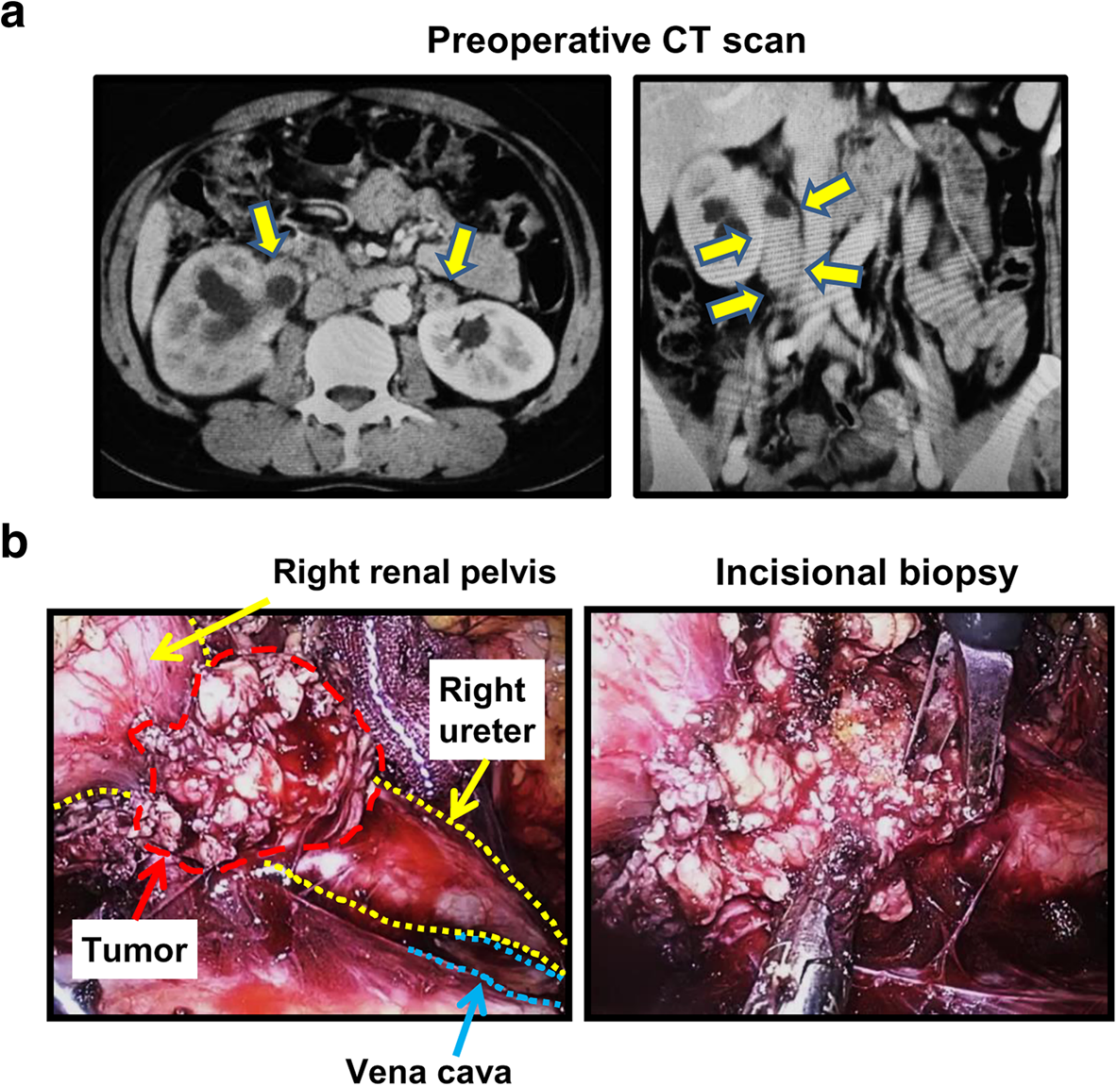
A representative case undergoing retroperitoneoscopic tissue biopsy. a Contrast-enhanced CT scan of a 39-year-old woman demonstrates extra-urinary tract lesions of bilateral ureters, which results in hydronephrosis. There are other multiple space-occupying lesions around the abdominal aorta and the inferior vena cava. b Intraoperative finding of the retroperitoneoscopic tissue biopsy. Tumors and their surrounding organs and structures are identified in order to make tissue biopsy easy and safe. A couple of tumor blocks are resected using cold scissors and removed through a small retrieval pouch. This case was pathologically diagnosed IgG4-related fibrosis
